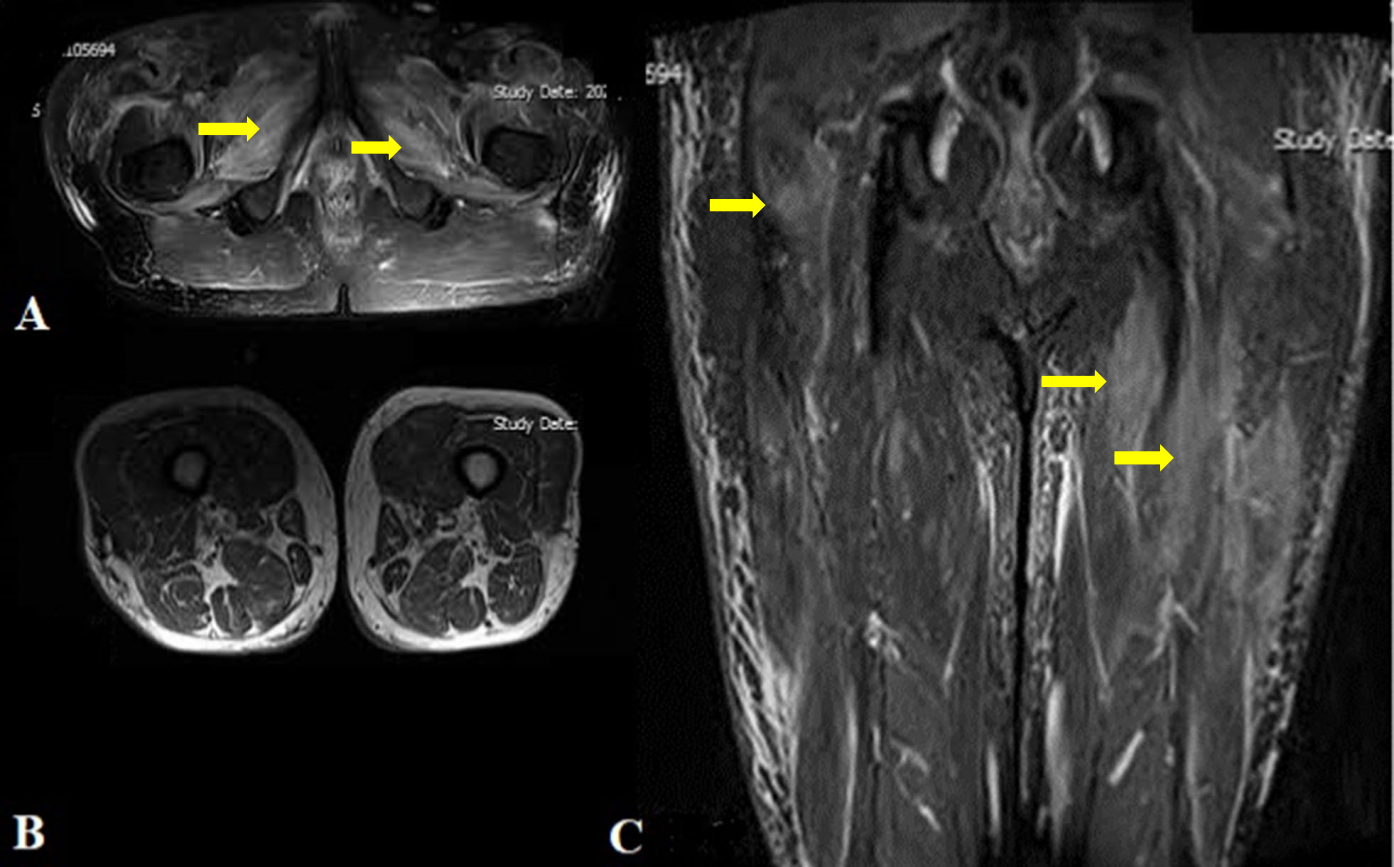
Bilateral thigh magnetic resonance imaging (without gadolinium contrast medium) T1 and T2 in axial and coronal view demonstrating diffuse edema within all muscle compartments of the thigh with notable intra- and perimuscular edema, suggestive of myositis; A axial T2; B axial T1; C coronal T1 (indicated with yellow pointer)
